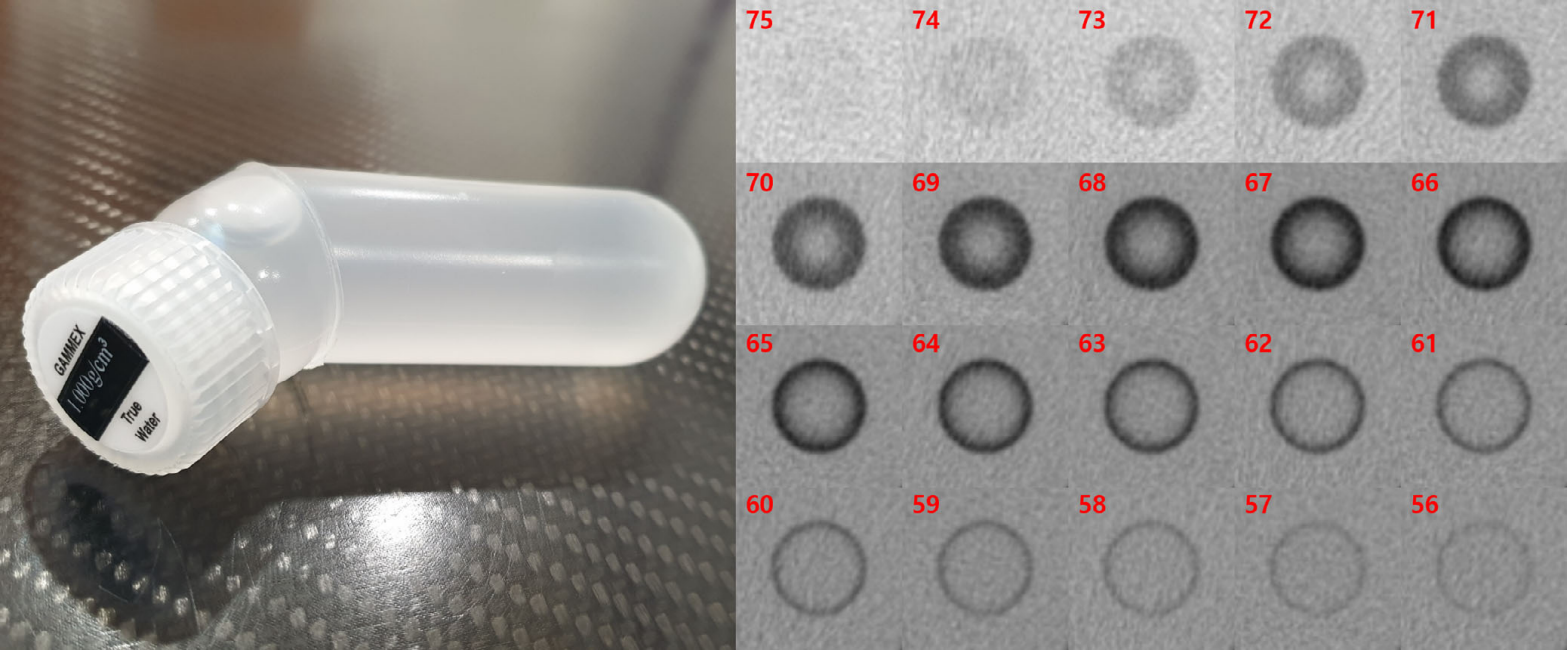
Liquid‐type container for true water (Gammex RMI, Middleton, WI) (left) and axial computed tomography images of the true water container according to slice number (right)
